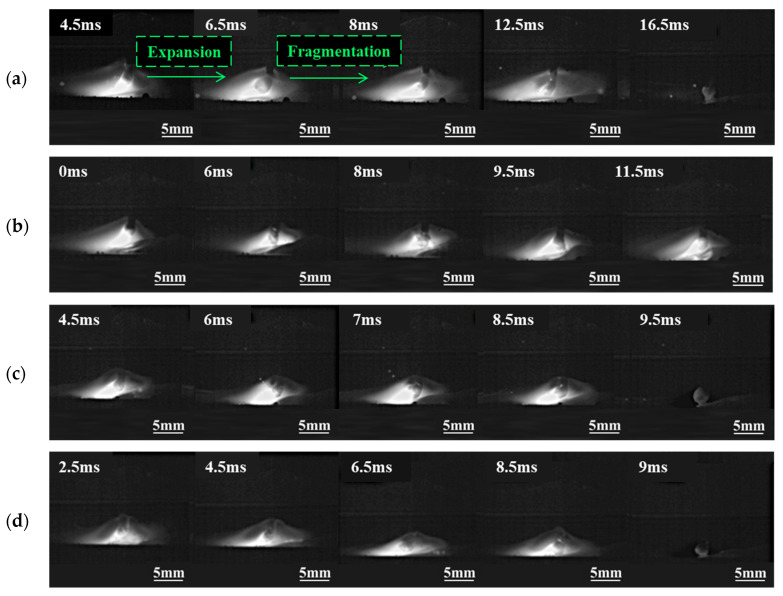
Influence of ultrasonic power on short circuit transfer. (a) 0.8 kW; (b) 1.2 kW; (c) 1.6 kW; (d) 2.0 kW.
